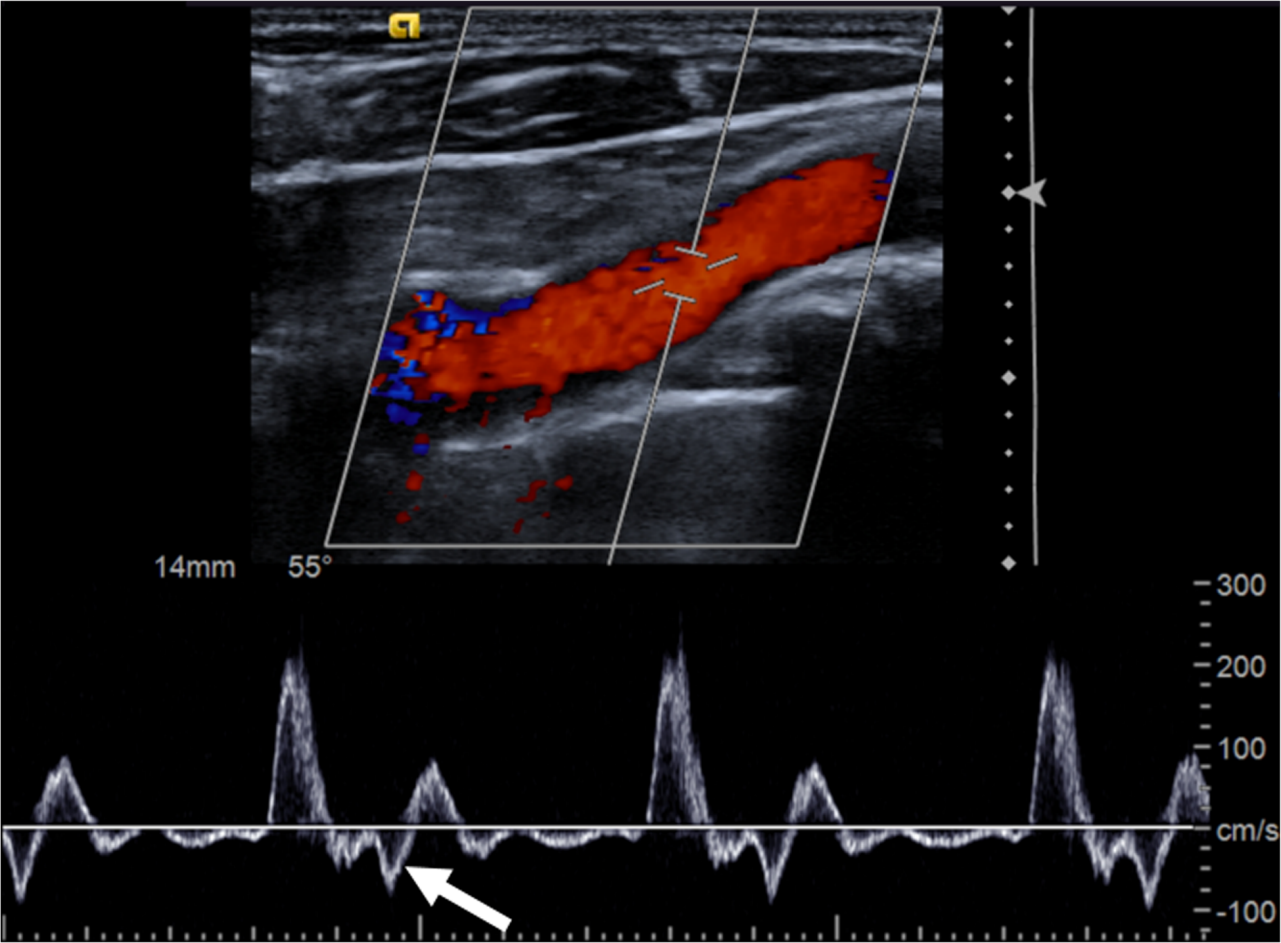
Doppler ultrasound of the right sub-clavian artery showing a retrograde flow (arrow)
